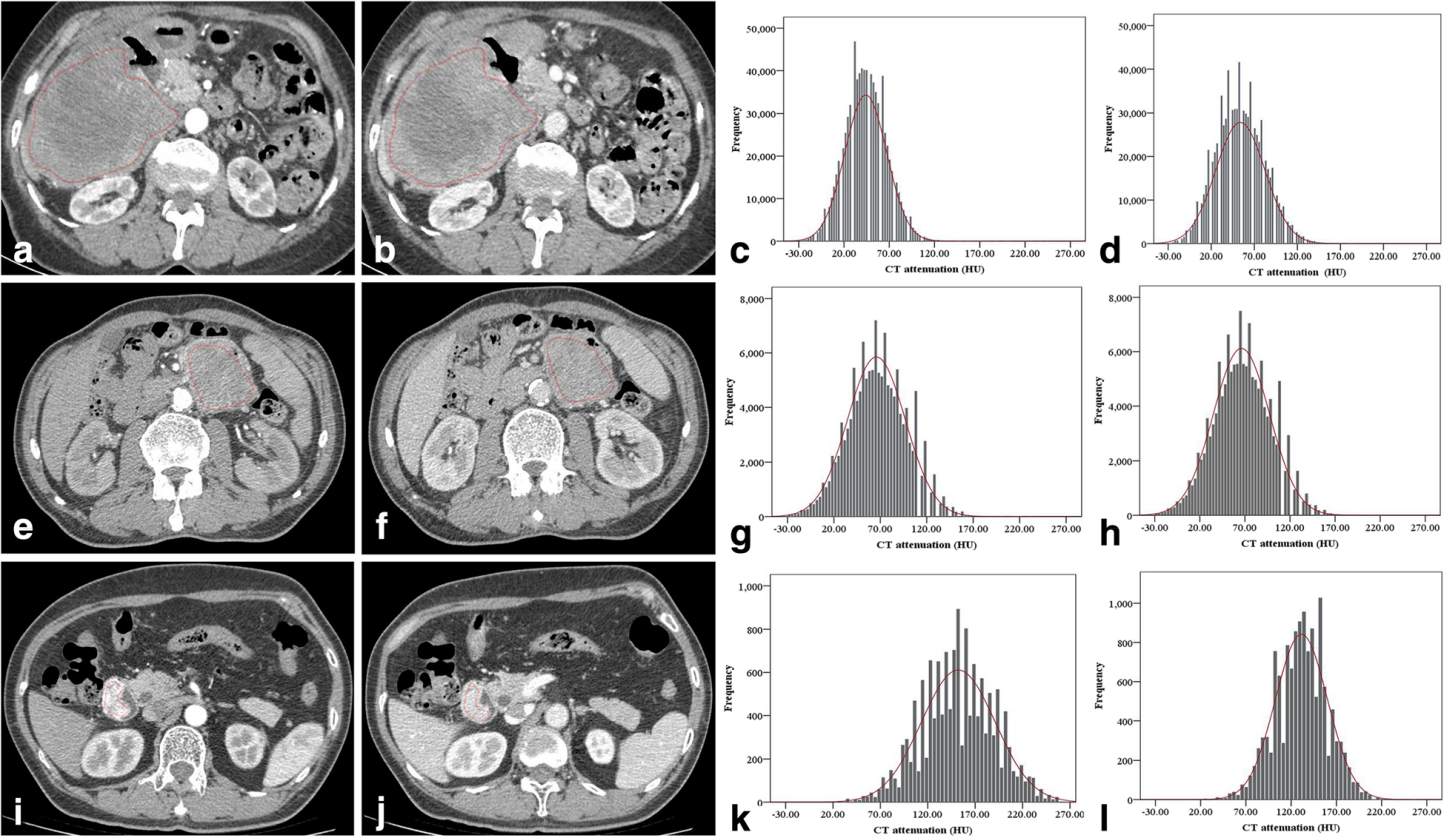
CT texture analysis of small bowel-GISTs with different risk levels. CT images of high risk in arterial phase (a) and venous phase (b) show an irregular external growth mass situated in the right upper abdomen with heterogeneous enhancement in a 66-year-old female. Histograms in arterial phase (c) and venous phase (d) show lower distribution of CT values. Mean attenuation and entropy were 47.00HU and 5.23 in arterial phase, 57.64HU and 5.39 in venous phase, respectively. CT images of intermediate risk in the arterial phase (e) and venous phase (f) show a rounded external mass at the proximal jejunum in a 60-year-old male. Histograms in arterial phase (g) and venous phase (h) show the distribution of CT values. Mean attenuation and entropy were 68.72HU and 5.31 in arterial phase, 70.22HU and 5.66 in venous phase, respectively. CT images of low risk in the arterial phase (i) and venous phase (j) show an irregular internal mass with obvious enhancement located at the descending duodenum in a 60-year-old female. Histograms in arterial phase (k) and venous phase (l) show higher distribution of CT values. Mean attenuation and entropy were 155.15HU and 6.21 in arterial phase, 133.26HU and 6.14 in venous phase, respectively
